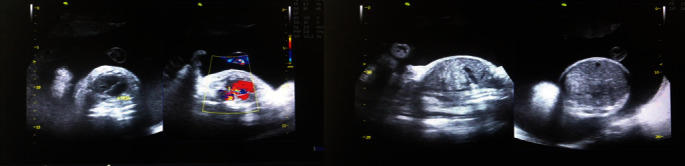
Sonograms showing small thoracic cavity with centrally placed heart, hypoplastic lungs, relatively large abdominal cavity, and increased liquor volume.
